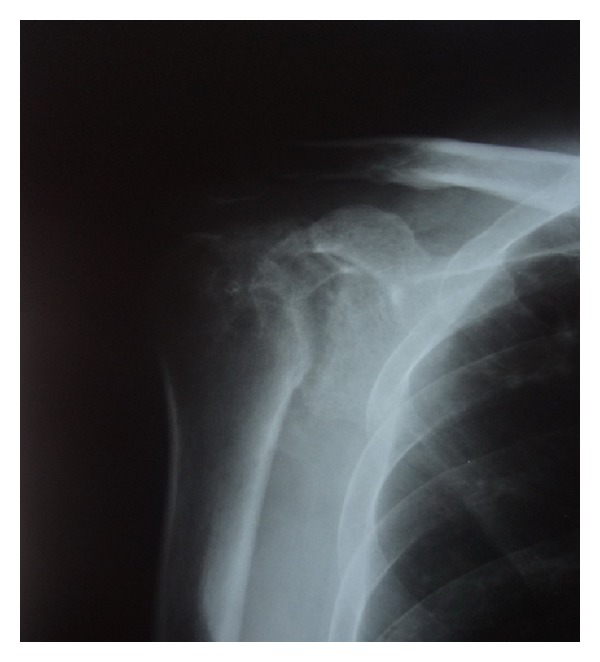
A plain radiograph showing severe glenohumeral joint destruction.
